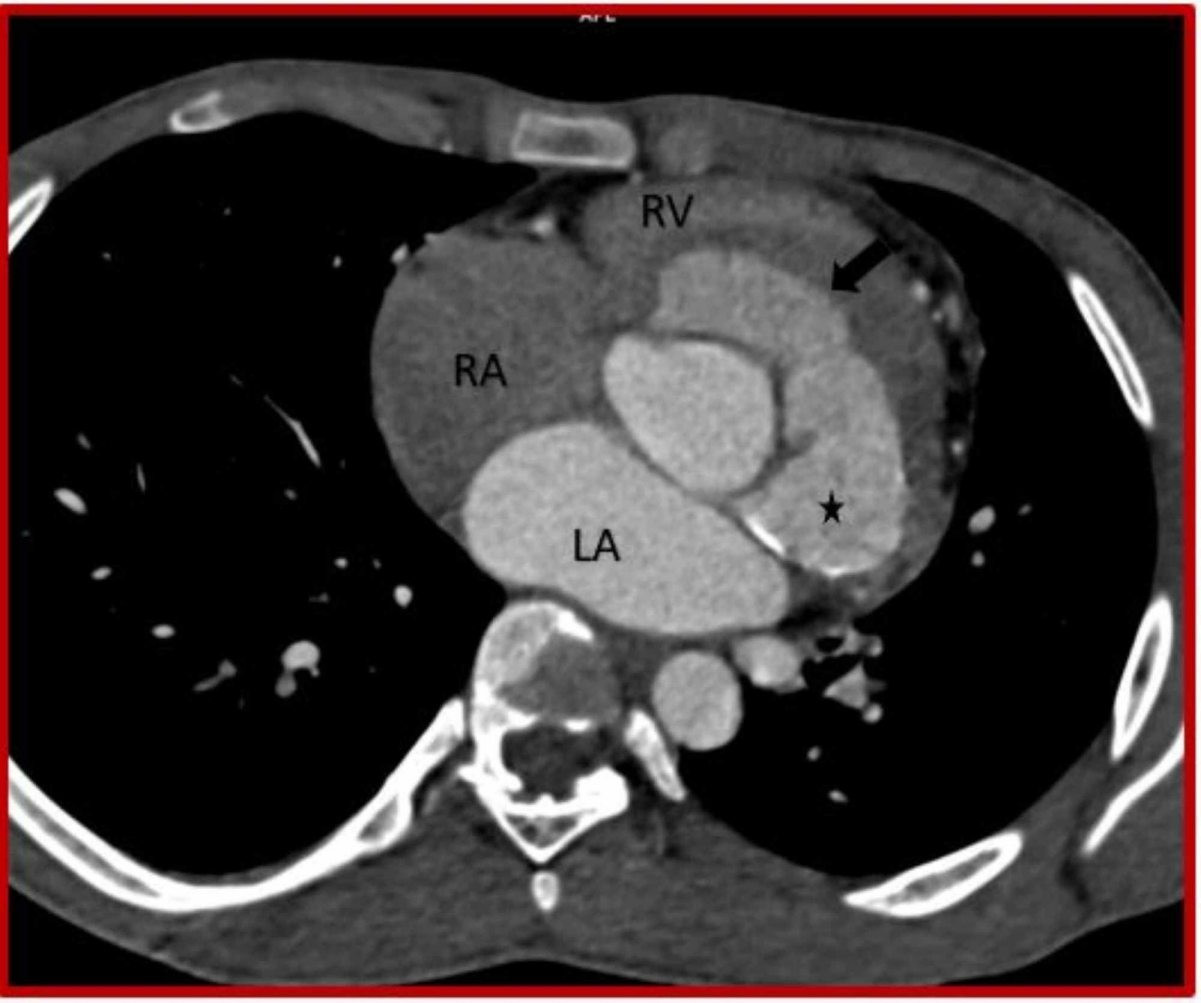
Cardiac CT (Axial view) demonstrates ruptured left sinus of Valsalva aneurysm (asterisk) dissecting into the interventricular septum (black arrow). CT: Computed tomography; RV: Right ventricle; RA: Right atrium; LA: Left atrium.
